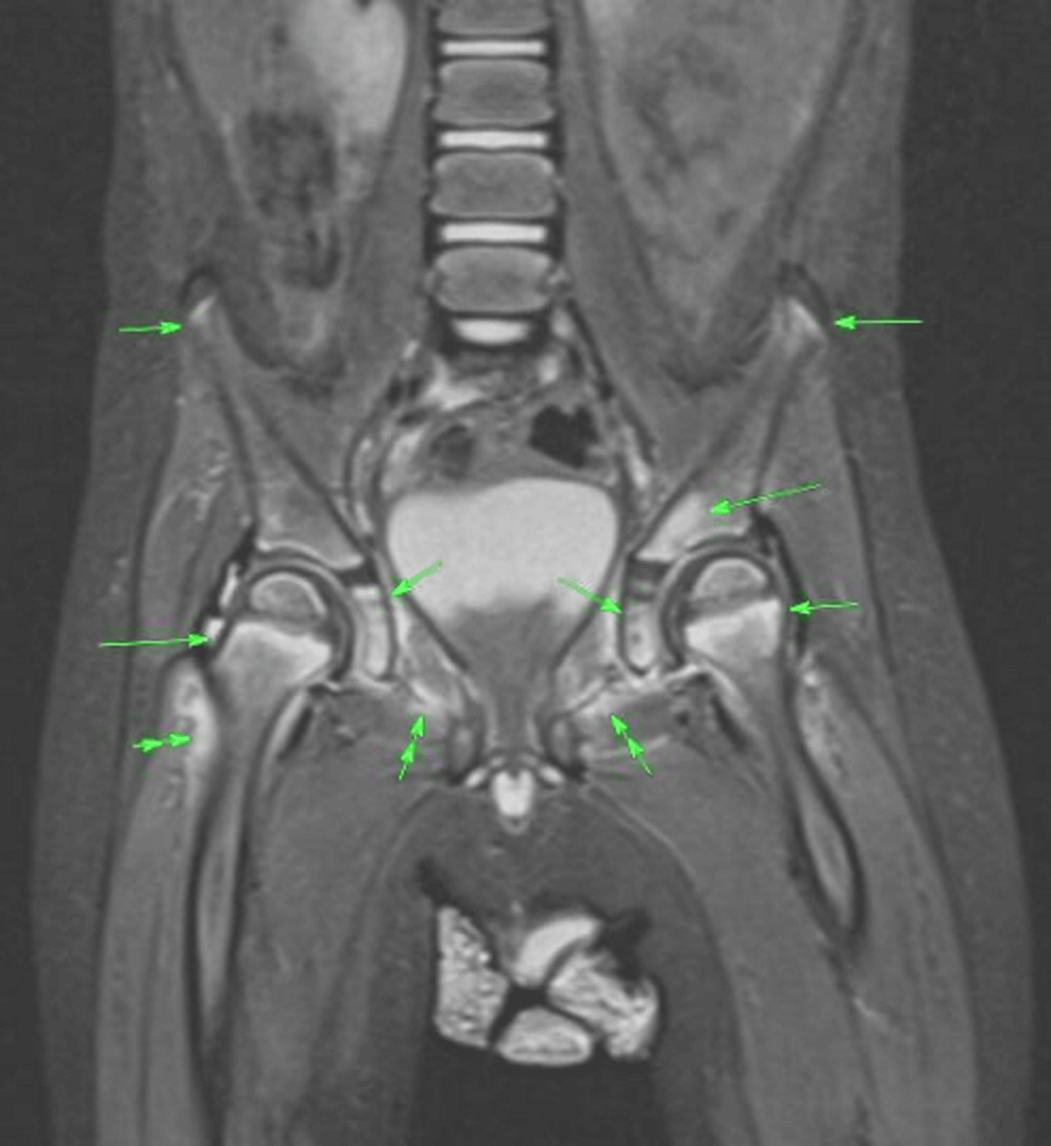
MRI STIR of bilateral hips. Symmetrical bone marrow oedema involving multiple metaphysis with associated peri-osseus soft tissue and muscle oedema are also noted. Area marked by single green arrows are bone oedema and double green arrows are muscular and soft tissue oedema. STIR: Short Tau Inversion Recovery
